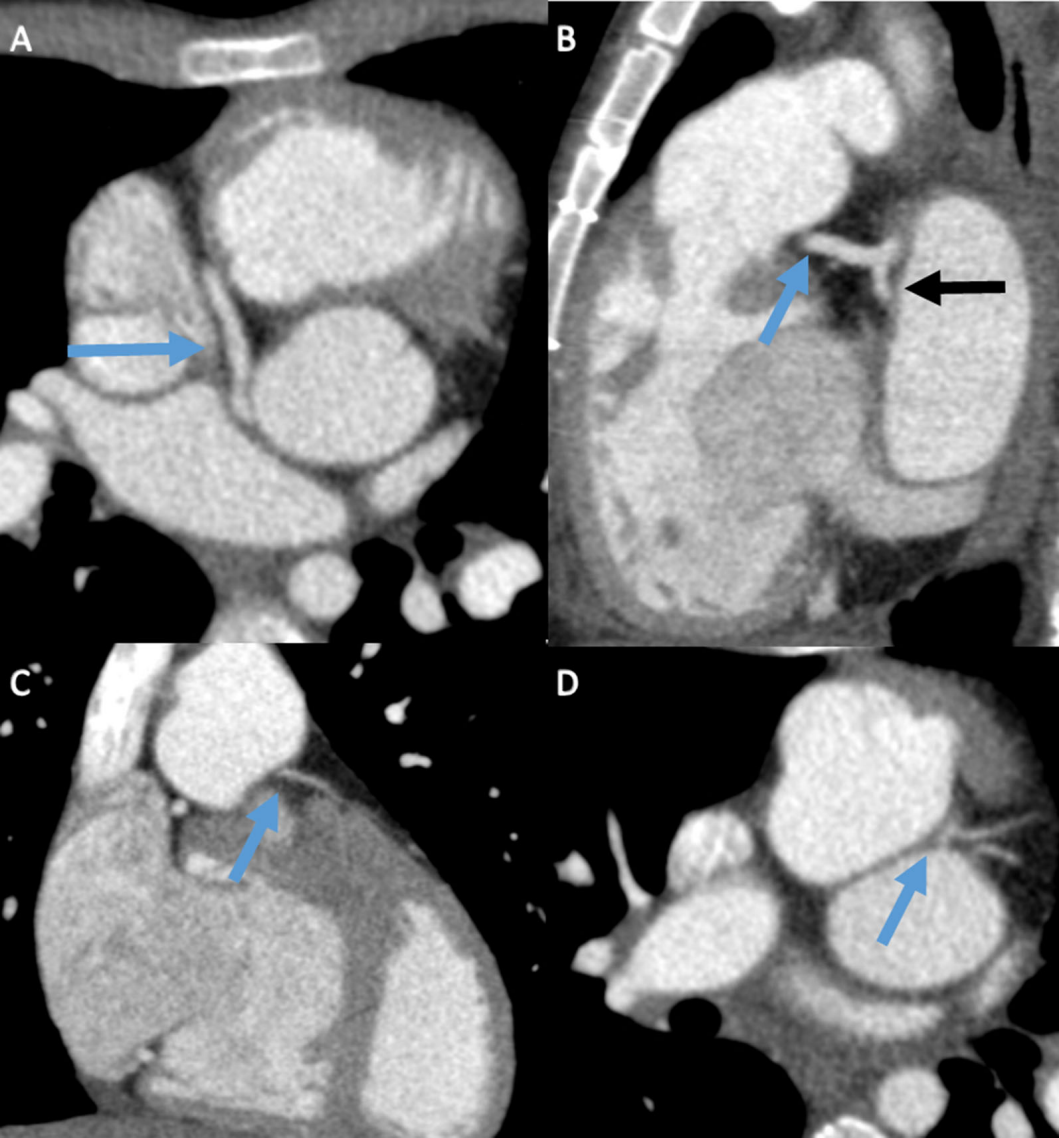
Cardiac CTA demonstrates filling of the RCA (A, blue arrow), an aberrant origin of the Cx arising from the RCA (B, black arrow). There is a fragile LAD (blue arrow) with suggestion of severe origin stenosis on coronal (C) and axial views (D)
